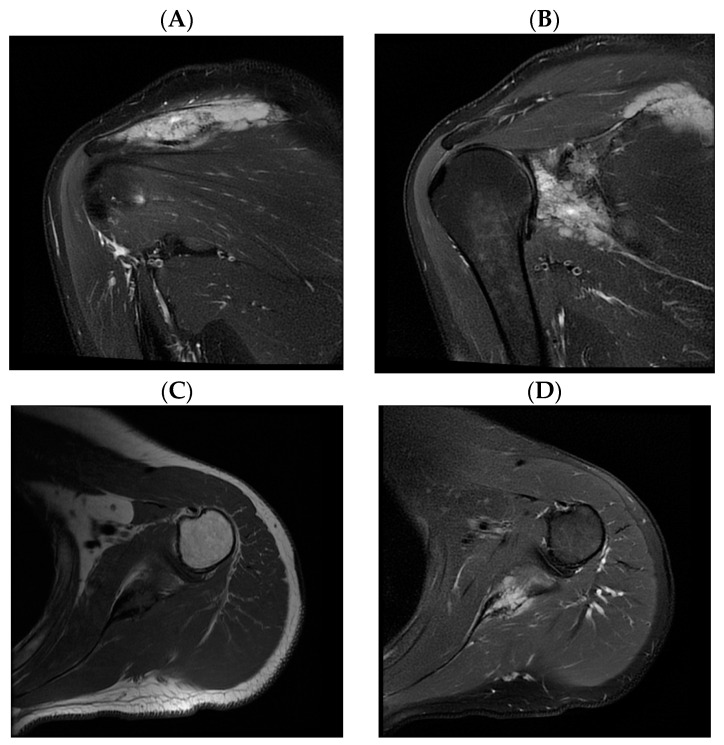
Magnetic resonance imaging (MRI) of the left shoulder. (A,B) Coronal short tau inversion recovery sequence images show extensive osteolytic, partly multilocular scapular lesions involving the lateral corpus, coracoid process, acromion, glenoid, and spina with periosteal reaction and destruction of cortical bone with extraosseous spread to surrounding soft tissue. No signs of infiltration of the surrounding muscles. (C) Axial T1-weighted and (D) axial proton density-weighted fat-saturated images show no signs of infiltration of the brachial plexus. The shortest distance between the distal part of the brachial plexus and the tumor is 33 mm. The rotator cuff tendons and the long head of biceps tendon are unremarkable. No signs of glenohumeral joint effusion or significant labral abnormalities.
